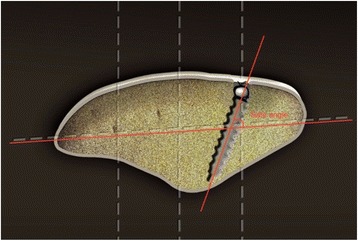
The *safe angle* was defined as the angle between the drilling tunnel and the connecting line. *Lines* connecting the upper-third horizontal plane and the medial and lateral margins of the patella were created on CT scans. The connecting line was divided into four parts. The area representing the medial one fourth of the patella surface was marked as the drilling site. The direction of the drilling referred to the safety angle according to patellar morphology
